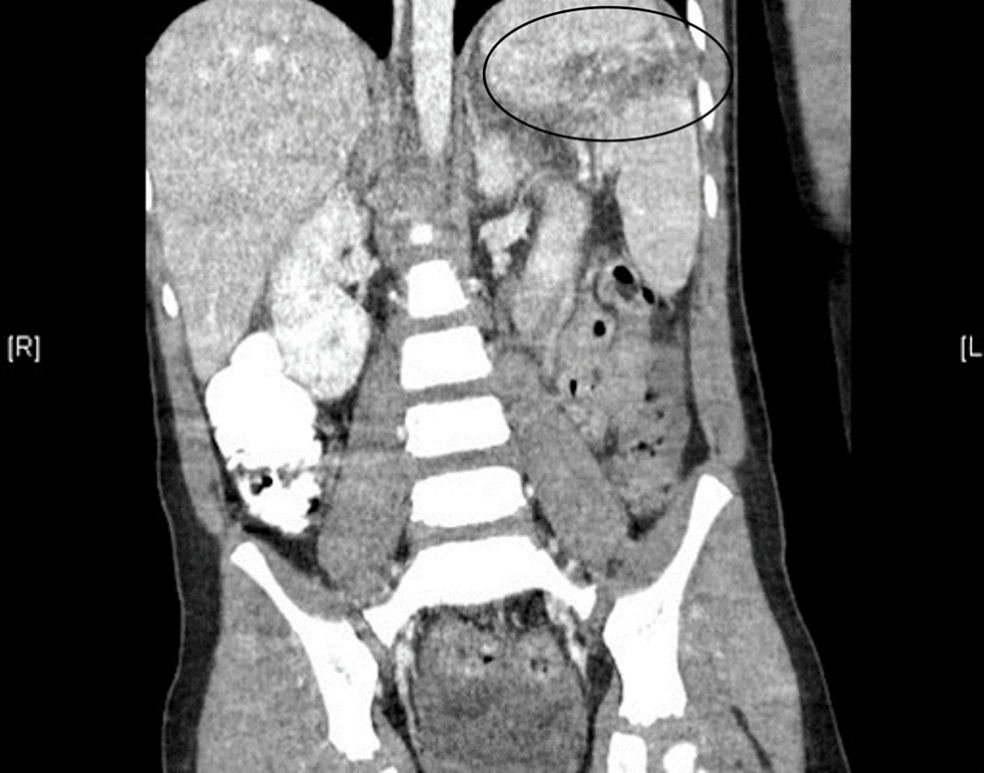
CT abdomen (coronal) consistent with splenic laceration
